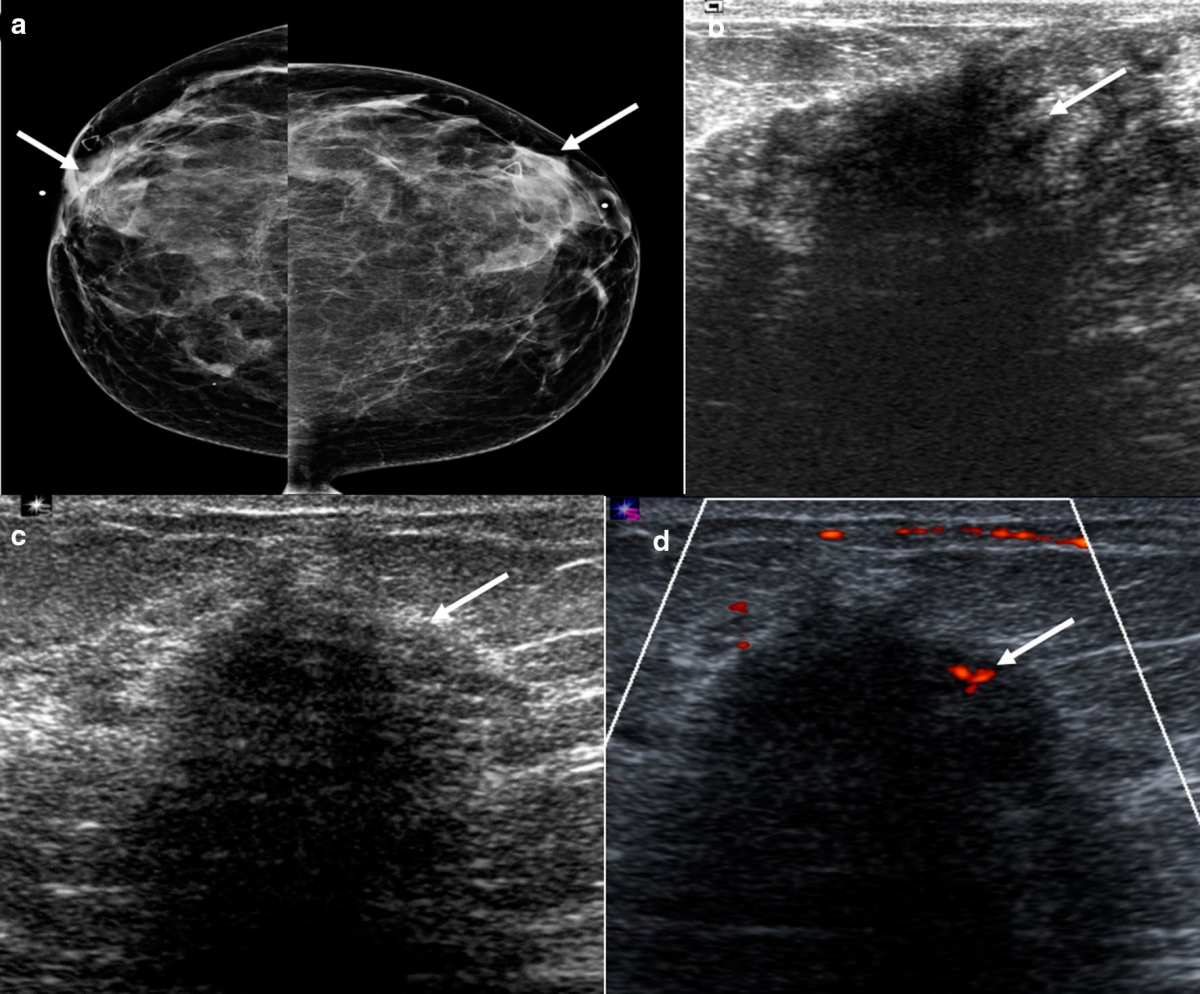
Diabetic mastopathy. A 56-year-old patient presented with bilateral palpable breast masses and an 8-year history of type 2 diabetes. Bilateral craniocaudal mammogram (a) shows bilateral non-calcified, obscured masses correlating with the palpable triangular markers (arrows). Grayscale right (b) and left (c) breast ultrasound shows irregular, hypoechoic masses with posterior acoustic shadowing (arrows). Power Doppler (d) ultrasound demonstrated internal vascularity (arrow) involving these masses. Core needle biopsy of both masses showed perilobular lymphocytic infiltration. Repeat core needle biopsy 8 months after the initial biopsy showed chronic lymphocytic lobulitis. No evidence of malignancy. Findings are consistent with diabetic mastopathy
